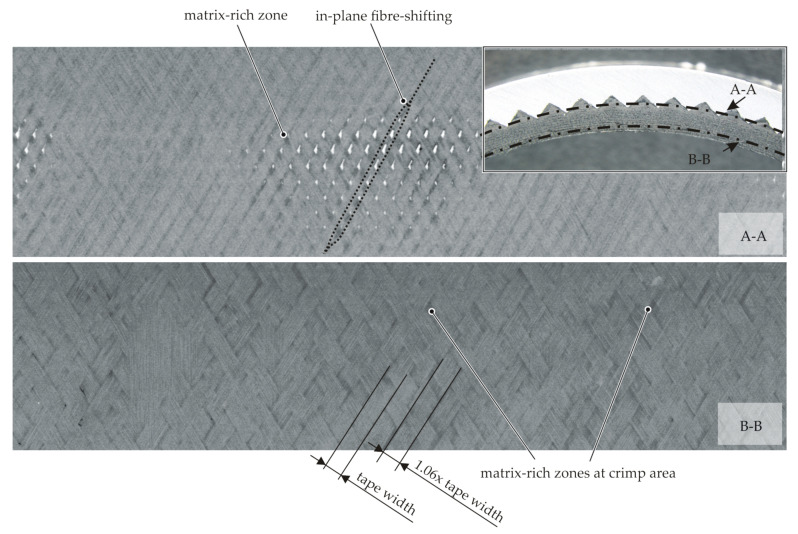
CT scan of the flattened surface of a meso-structured contour joint.
